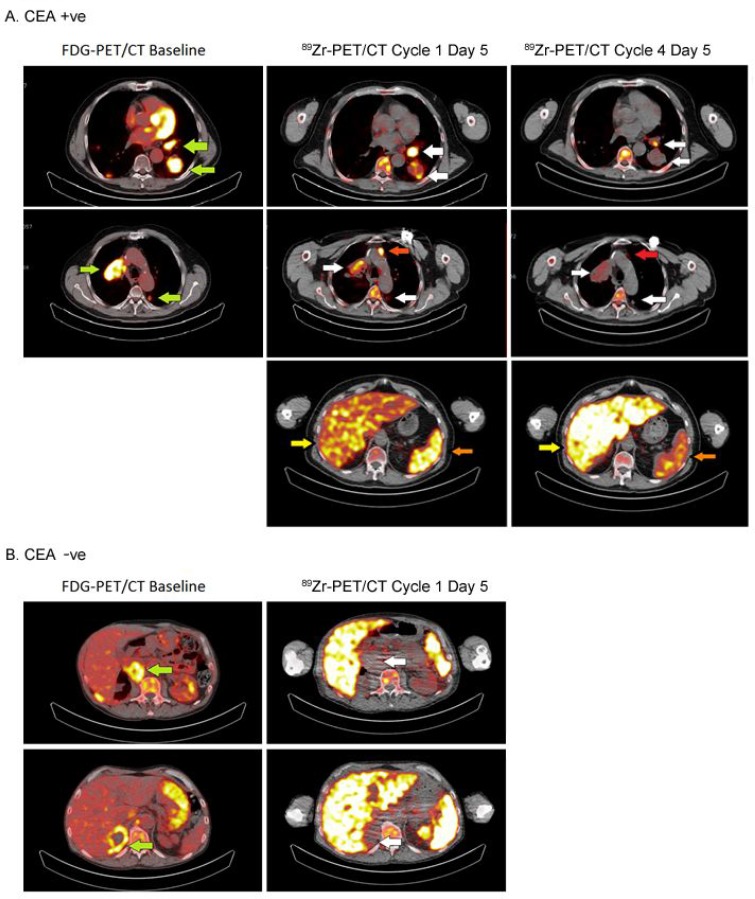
Tumor accumulation in a CEA+ and a CEA− patient treated with 20 mg and 30 mg of CEA-IL2v, respectively Images of ^89^Zr-PET at cycle 1, day 5 (96 hours) are shown (left) and tumor lesions were identified by ^18^F-FDG-PET at screening (right, green arrows). In the patient with CEA+ colorectal cancer (A), ^89^Zr-CEA-IL2v accumulation was observed in the left and right hilar lymph nodes, the left dorsal lung lesion (accumulation indicated by white arrows) and a non-pathological lymph node (accumulation indicated by red arrow) in the CEA+ patient. For the CEA− patient (B), the adrenal gland and abdominal lymph node lesions were negative on ^89^Zr-PET. Part A also shows that accumulation of ^89^Zr-CEA-IL2v in tumor decreased between day 5 (96 hours) of cycle 1 and cycle 4. Following the first dose of 20 mg, this patient received 30 mg every 2 weeks for subsequent cycles as part of cohort E. Also spleen accumulation was decreased (orange arrow), while liver accumulation was increased (yellow arrow).
